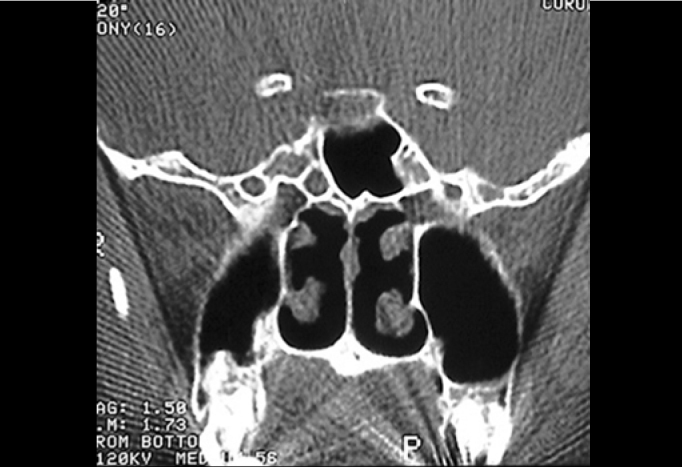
Computed Tomography of patient with CSF fistula in meningocele of sphenoid sinus.
